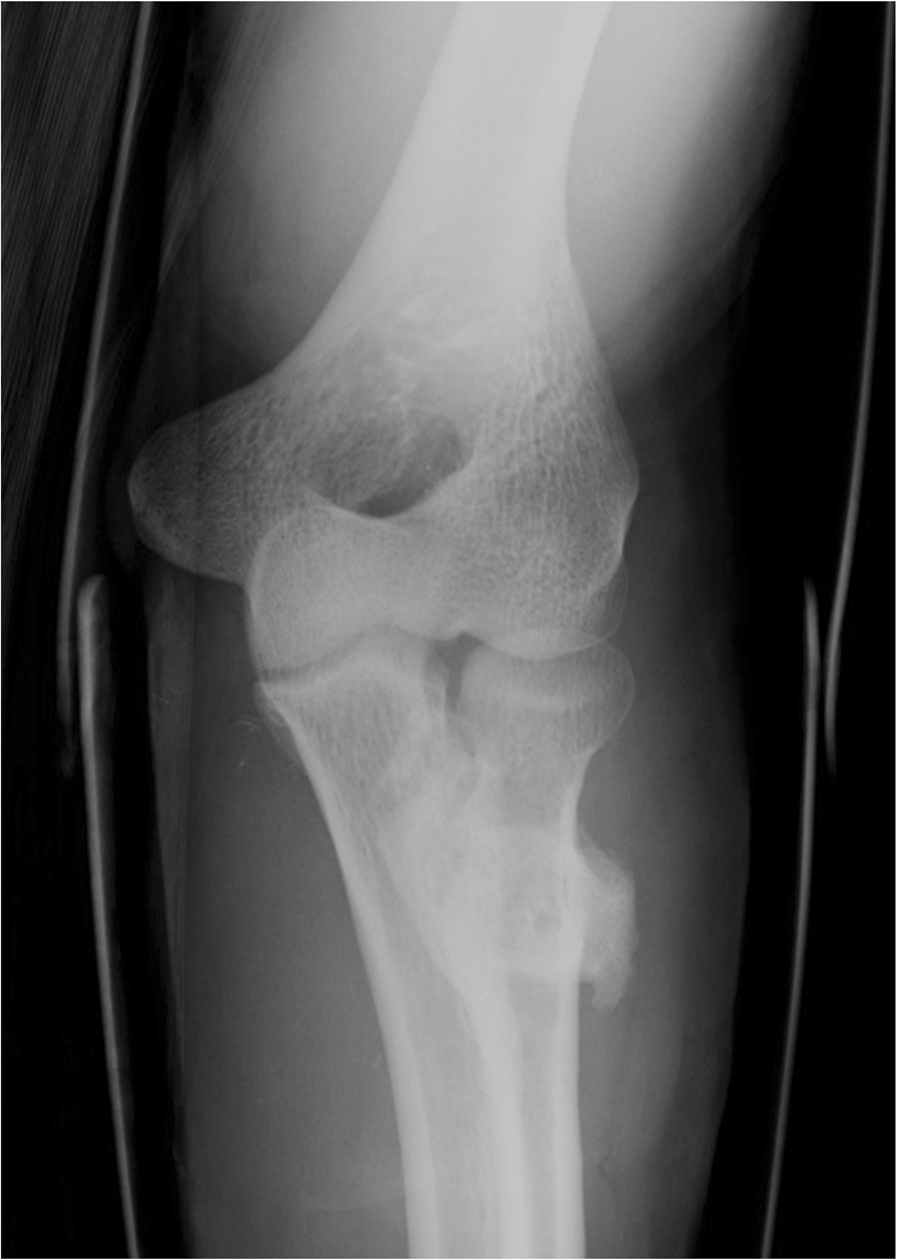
A case of proximal radio-ulnar synostosis with radiographic documentation
